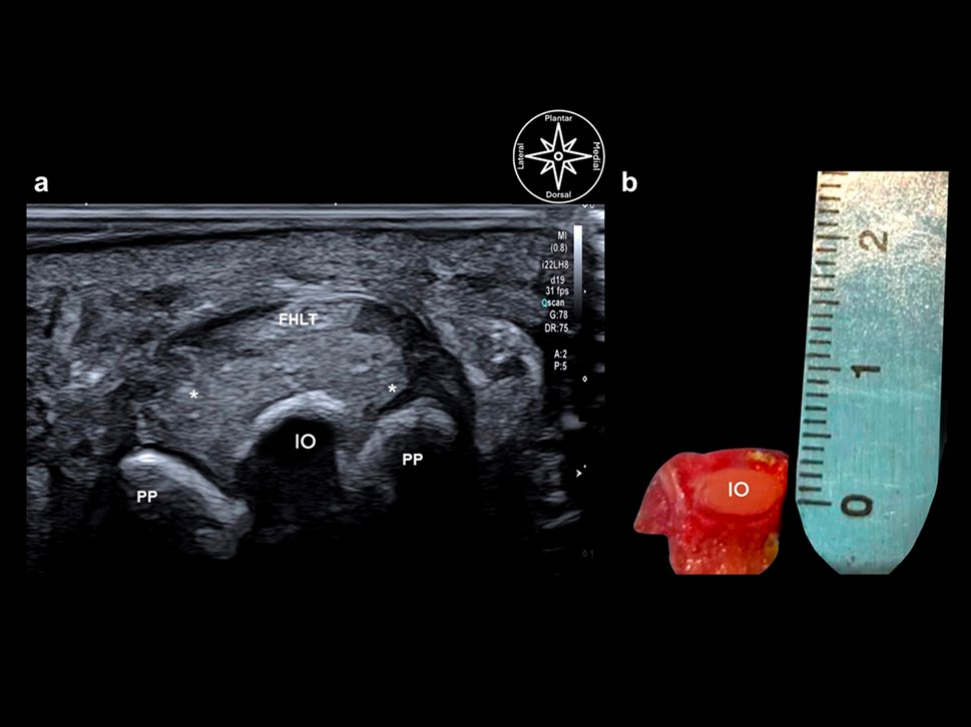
Comparison between diagnostic ultrasound (a) and gross anatomy of the interphalangeal ossicle in the axial view (short axis). (b) *PP* proximal phalanx, *IO* interphalangeal ossicle (5.8 mm), *FHLT* flexor hallucis longus tendon, asterix* plantar plate, *IO* interphalangeal ossicle (6 mm).
